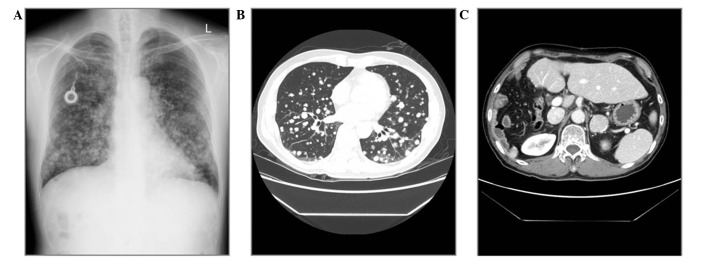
(A) Chest radiography and computed tomography scans of the (B) chest and (C) abdominopelvis in February, 2009 revealed multiple metastatic nodules in the two lung fields, bilateral adrenal metastases and a metastatic lymph node ~1.6 cm in size adjacent to the liver resection margin.
